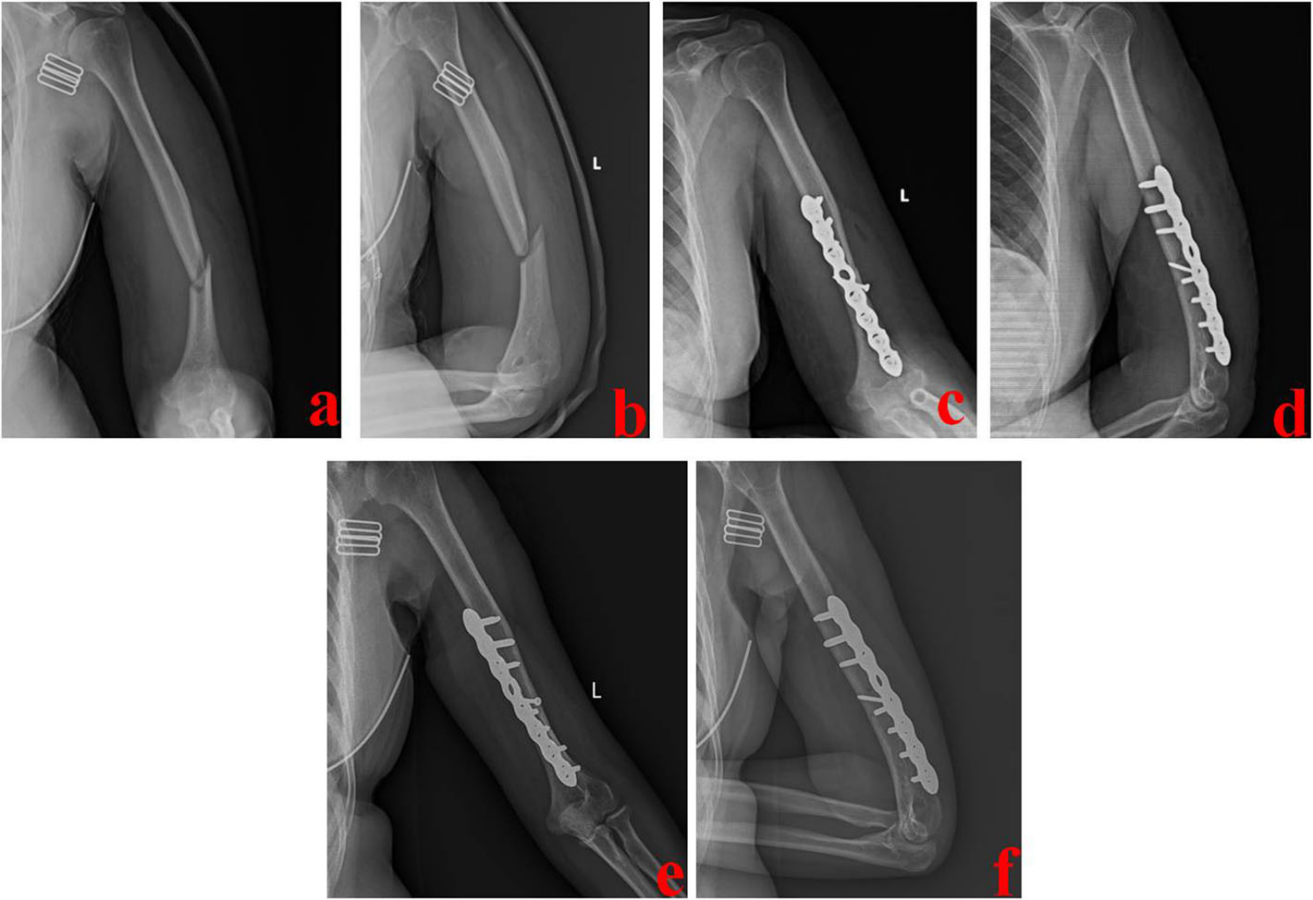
A 42-year-old female was treated by PMA technique. a and b Preoperative X-ray films showed a middle and distal-third humeral shaft fracture. c and d Immediate postoperative X-ray films showed good reduction and fixation. e and f One year after operation, X-ray films showed that this fracture healed well. PMA stands for the posterior median approach
